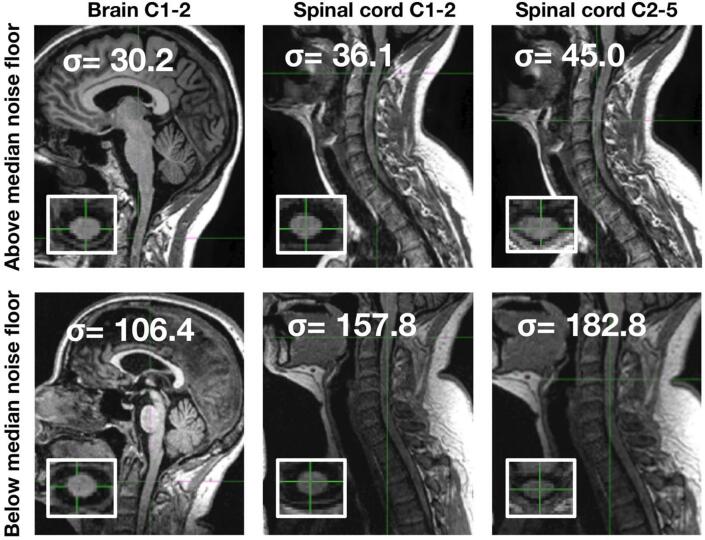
MRI noise floor. Figure shows sagittal plane of brain and spinal cord images above (upper row) and below (lower row) the median noise floor (axial plane in the inset). Different spinal cord levels are reported (C1-2 from brain MRI, C1-2 from spinal cord MRI, and C2-5 from spinal cord MRI). Standard deviation of the MRI signal (σ) within the CSF surrounding the spinal cord is reported for each image. CSA: cross-sectional spinal cord area; GBSI: generalised boundary-shift integral; MRI: magnetic resonance imaging.
